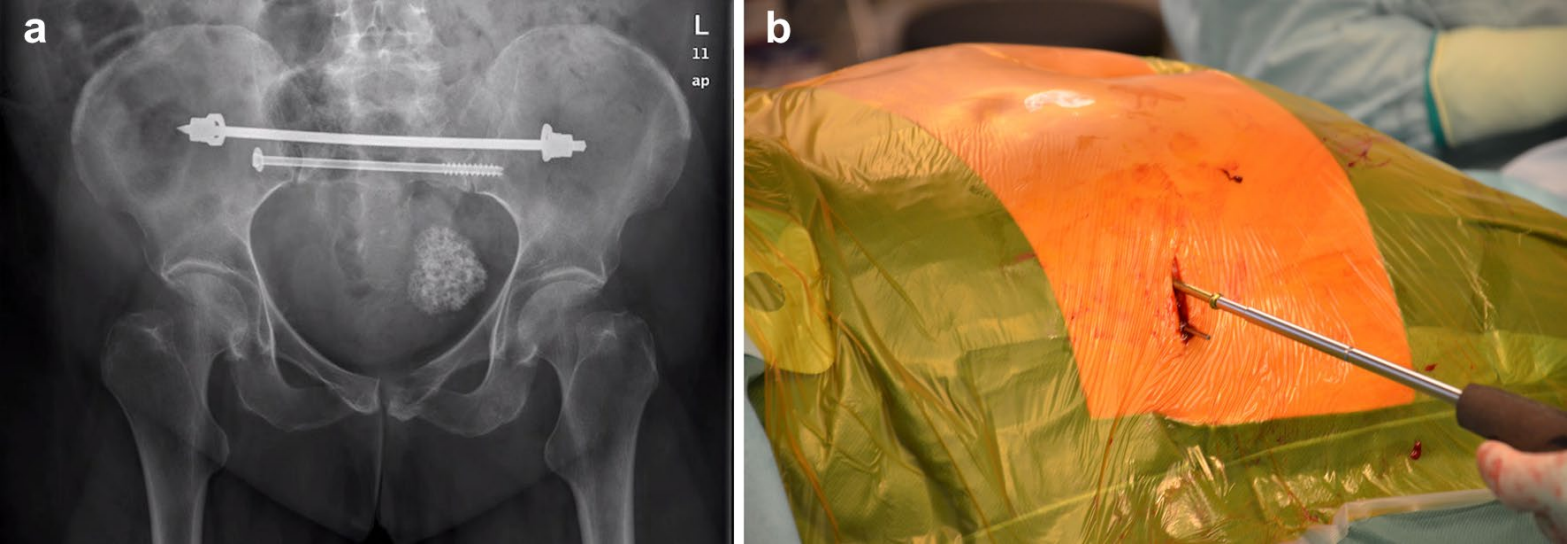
a and b X-ray pelvic overview (a) and intraoperative image (b) of a bisegmental transsacral screw fixation
